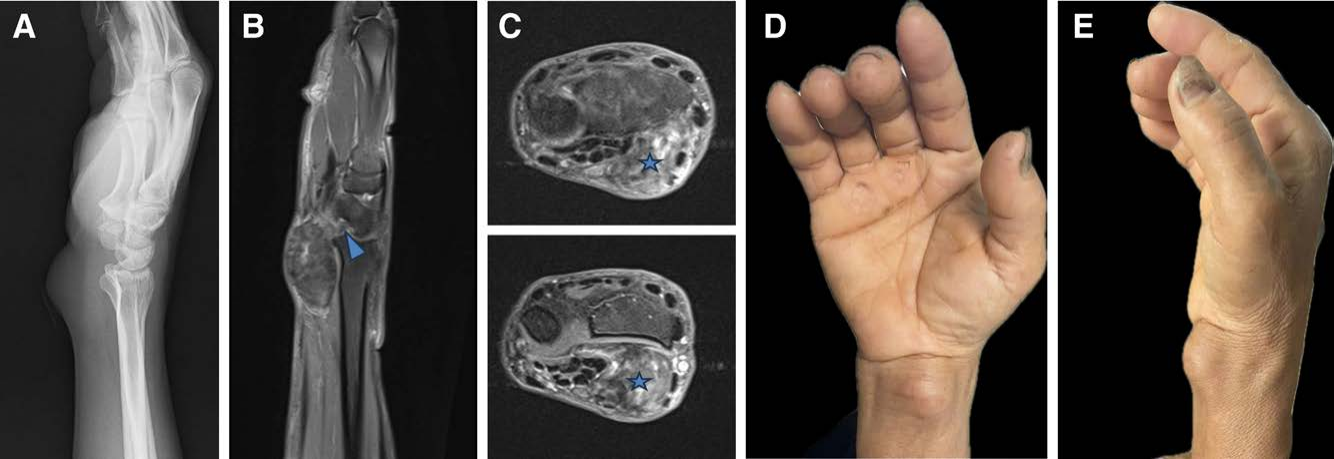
Preoperative clinical and imaging examination. (A) Lateral radiograph of the wrist showing subtle scalloping of the volar aspect of the distal radius. (B) Sagittal T2/PD-weighted MRI demonstrating a heterogeneous and slightly hypointense mass at the wrist, extending into the radiocarpal joint (arrowhead). (C) The tumor (star) displaces adjacent structures medially. (D, E) Anteroposterior and lateral views of the tumor. MRI = magnetic resonance imaging, PD = proton density.
